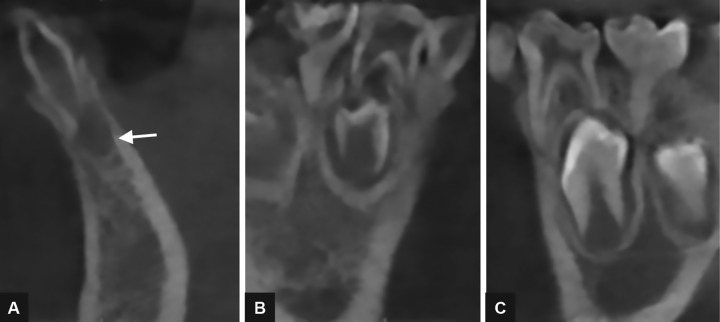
Transverse images of CBCT showing the tooth 82 with a “ghost tooth” aspect associated with a hypodense image (white arrow) suggestive of periapical lesion (A); in addition, a significant difference between the space of the pulp chambers of primary teeth 84 (B) and 74 (C)
